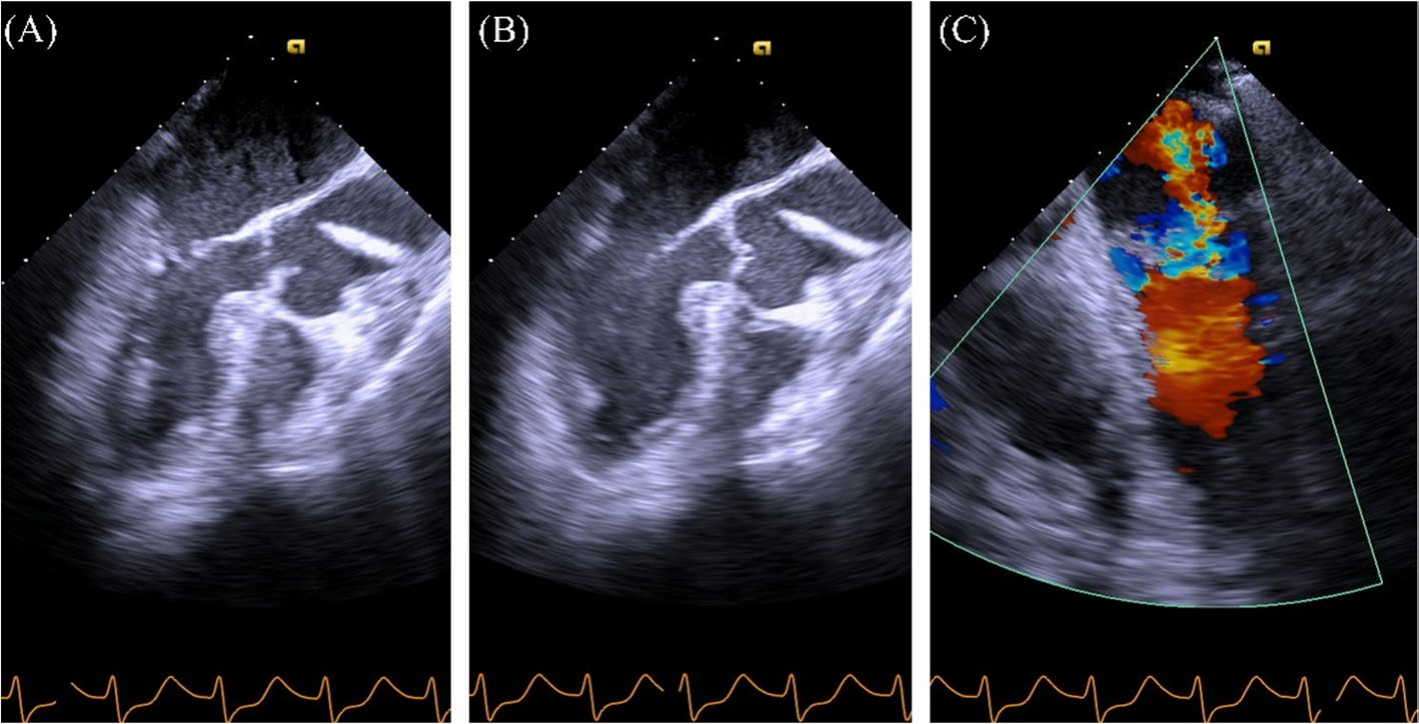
Intraoperative transesophageal echocardiography(TEE). A, B TEE images showing severe hypokinesis of the anteroseptal wall in A (systole) and B (diastole) (midesophageal long-axis view). C A TEE image with the application of color Doppler showing severe mitral regurgitation (midesophageal four-chamber view)
